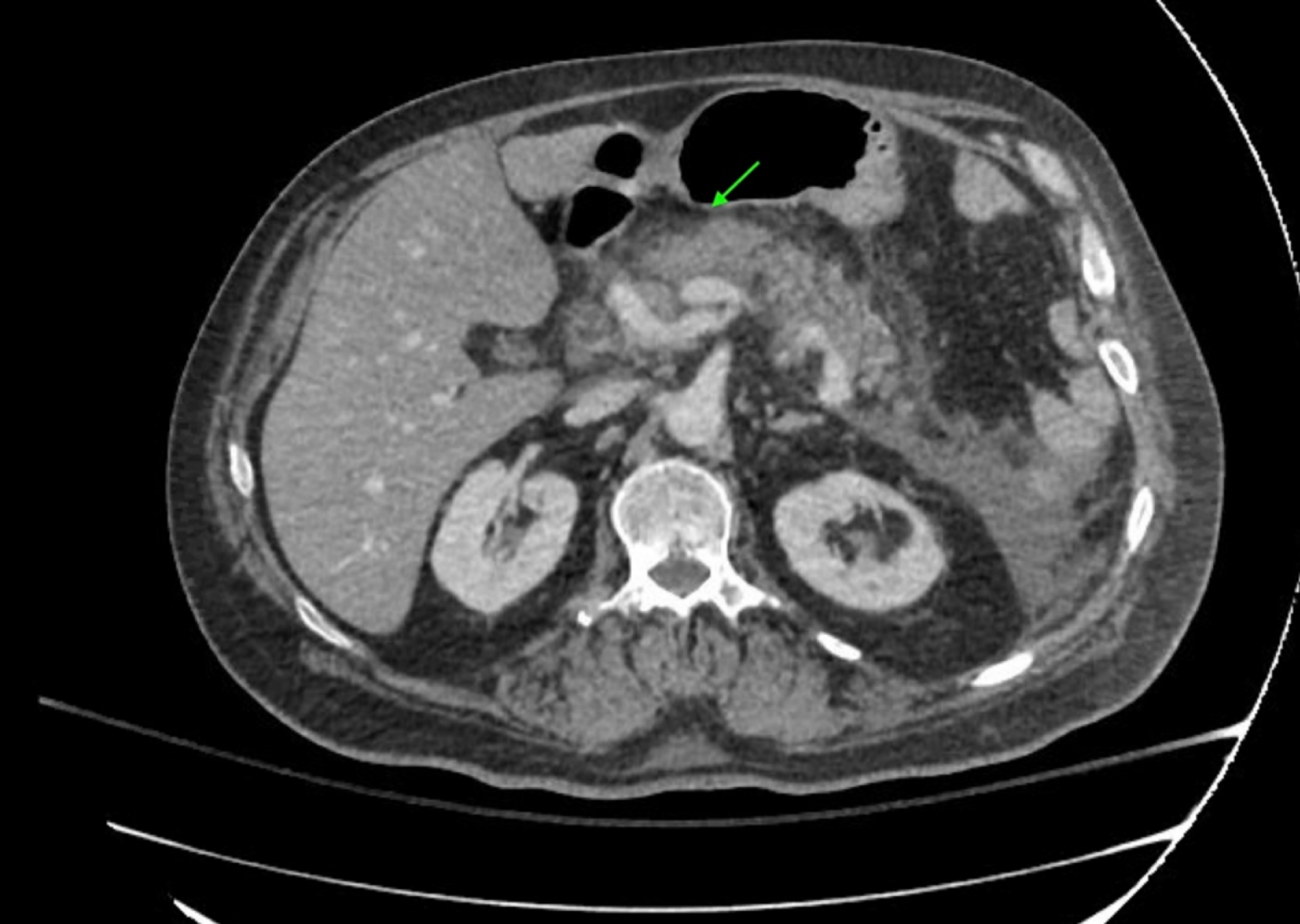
Computed tomography (CT) finding of acute pancreatitis Arrow depicts peripancreatic edema.
